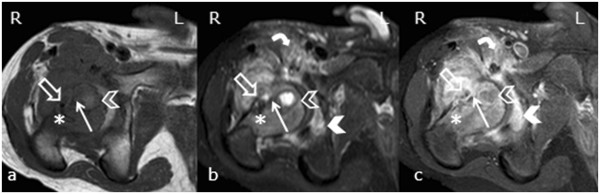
Magnetic resonance imaging of the right hip with axial T1-weighted sequences (A), T2-weighted sequences using a fat suppression signal (B) and T1-weighted sequences after an intravenous injection of paramagnetic contrast medium (C), showing an area of T1 hypointensity and T2 hyperintensity in the meta-diaphyseal region of the femur with diffuse enhancement via the paramagnetic contrast medium (arrow) and a peri-osteal reaction on the medial face (open arrow). Joint effusion and synovitis (closed arrowhead) are evident. The surgical path (*) can be observed in the lateral margin of the proximal femoral metaphysis for the biopsy site. Intra-osseous collection in the meta-diaphyseal region (open arrowhead) and edema of the iliopsoas muscle belly (curved arrow) are also visible.
